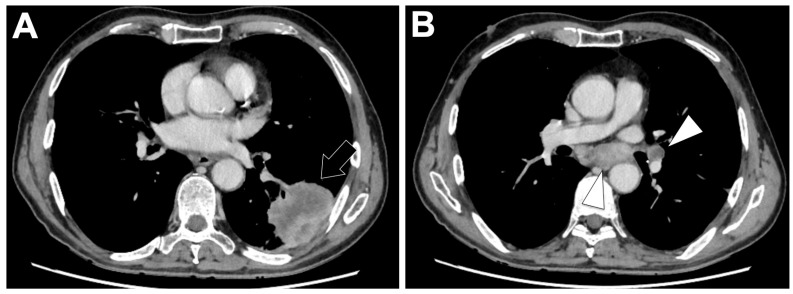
Representative baseline CT imaging findings in patients with MET exon 14 skipping lung adenocarcinoma (LUAD). A 76-year-old man with a history of tobacco use presented with a large, necrotic, peripheral, lobulated mass in the left lower lobe (black arrow) (A) accompanied by multiple necrotic mediastinal lymphadenopathies (white arrowheads) (B).
